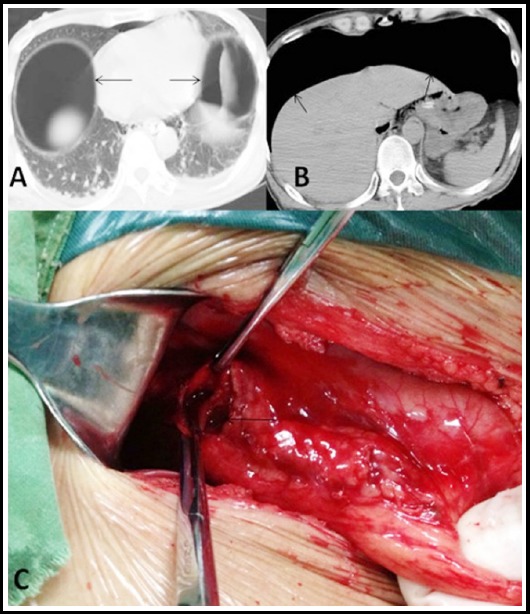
Computed tomography and intraoperative photograph of a patient with gastric perforation after improper cardiopulmonary resuscitation. A and B: Chest and abdomen CT scan show an appearance of gas (arrow) in the abdomen and the contractible stomach. C: A 2-cm laceration (arrow) is seen at the lesser curvature of the stomach.
